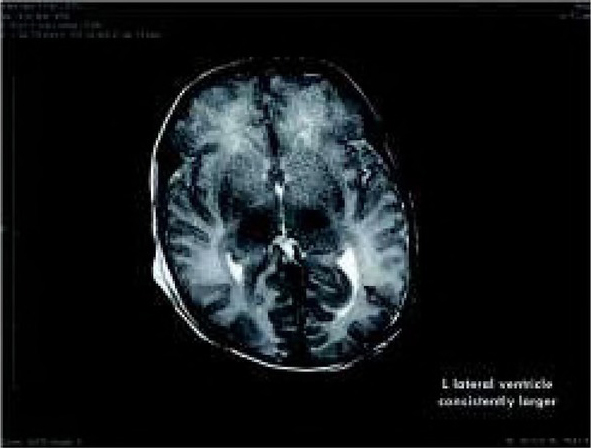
Brain MRI of the patient, arrow points to the affected left side. Ryc. 5. MRI mózgu pacjenta, strzałka wskazuje na uszkodzoną lewą stronę.
